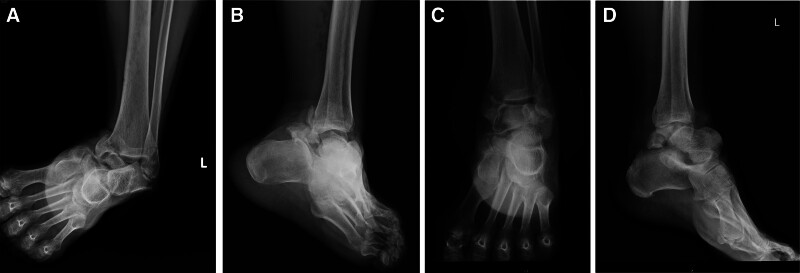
AP and lateral radiograph of the left ankle demonstrating fracture and dislocation of the talus with tri-malleolar fracture (A, B) and after reduction (C, D).
